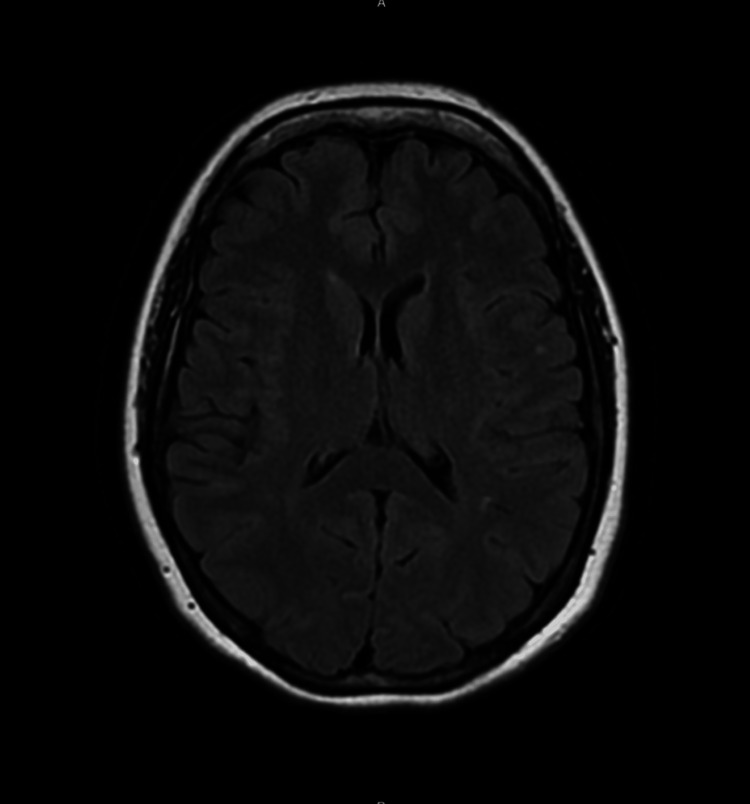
Axial T2-FLAIR MRI showing mild periventricular white matter changes and two punctate hyperintense lesions in the left hemispheric region. FLAIR: fluid attenuated inversion recovery
